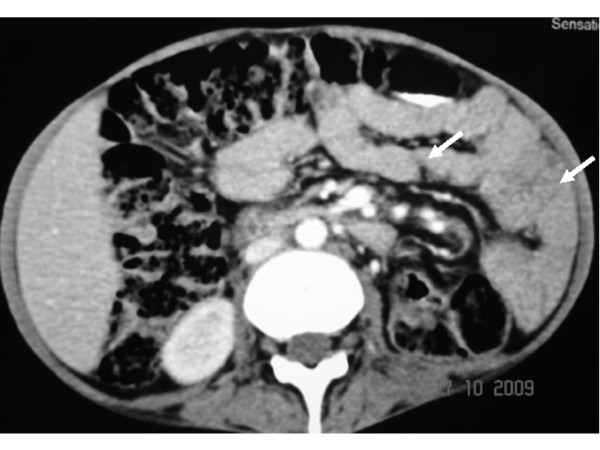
Axial contrast enhanced CT image shows diffuse, concentric wall thickening of multiple small bowel loops (arrows).
